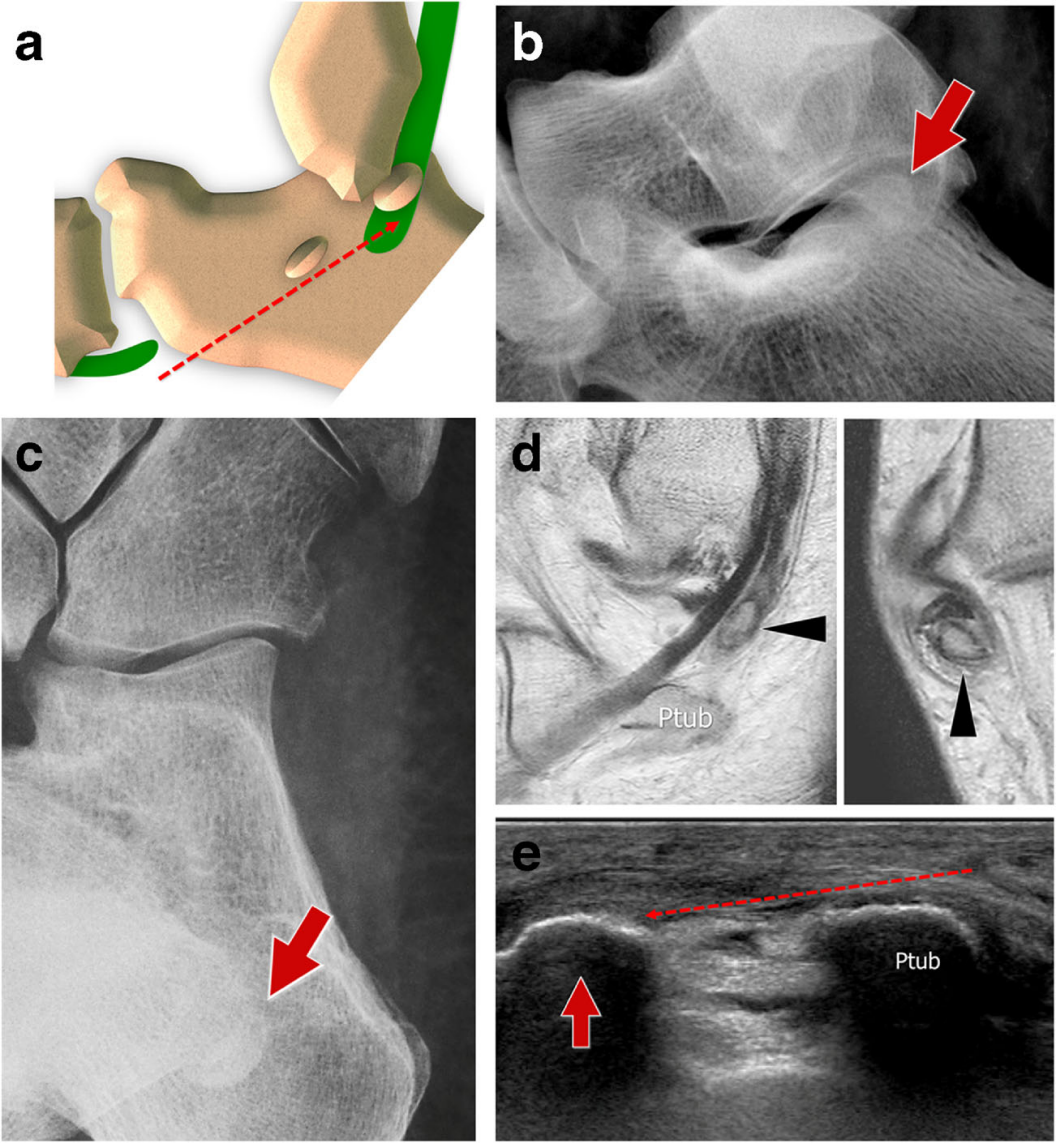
PLT tear type III proximal retraction. *15A* Scheme: proximal retraction after strong contraction. *15B–C* Lateral and internal oblique radiograph. The OP (*arrows*) can be found at the level of the posterior subtalar joint. *15D–E* Magnetic resonance imaging and ultrasound. The OP (*arrows*) can be found posteriorly to the PTub
